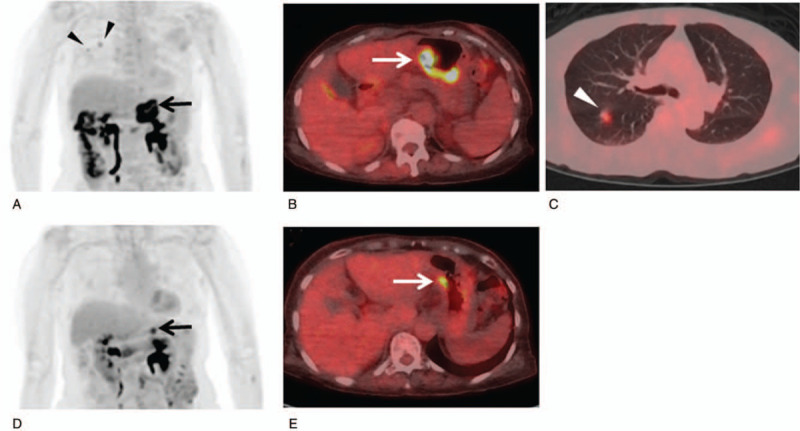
A 74-year-old woman with advanced gastric cancer with lung metastases (stage IV) received nivolumab. Baseline ^18^F-FDG-PET/CT [maximum intensity projection (MIP) (A) and fused transaxial (B and C) images] shows abnormal ^18^F-FDG uptake in the primary lesion (A and B: arrows) and right lung metastases (A and C: arrowheads). The follow-up ^18^F-FDG-PET/CT after 4 courses of nivolumab therapy [MIP (D) and fused transaxial (E) images] show decreases of ^18^F-FDG uptake in the primary lesion (D and E: arrows) with disappearance of lung metastases. The reductions of the sum of SUVmax and SULpeak were 72.9% (15.76–4.27) and 72.2% (9.46–2.63), respectively. The status was PMR according to EORTC or PERCIST. The patient was alive without progression 26 mo after the initiation of nivolumab.
